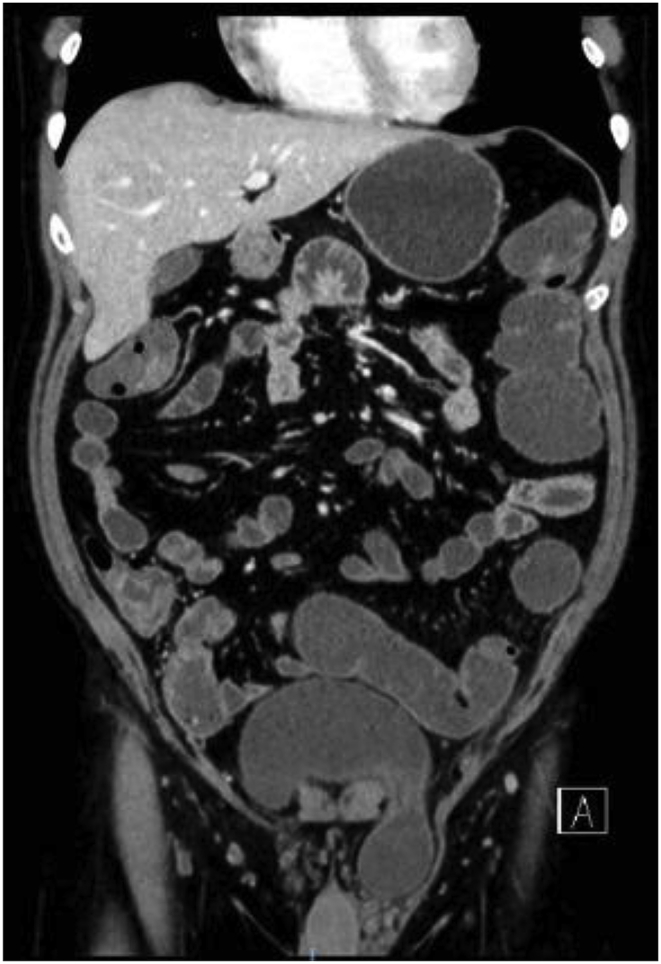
CT scan of the abdomen and pelvis showing bilateral vesical inguinal hernia, more prominent in the left side (Coronal View).
